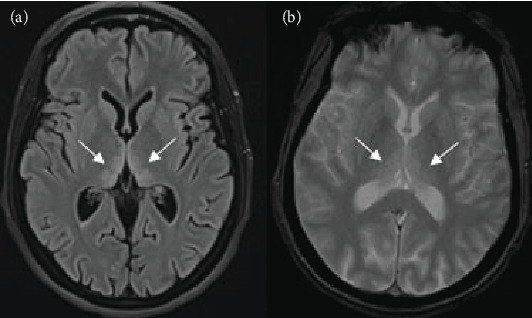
MRI showing hypersignal lesions (arrows) in the thalami on axial FLAIR (a) and T2-weighted (b) sequences.
